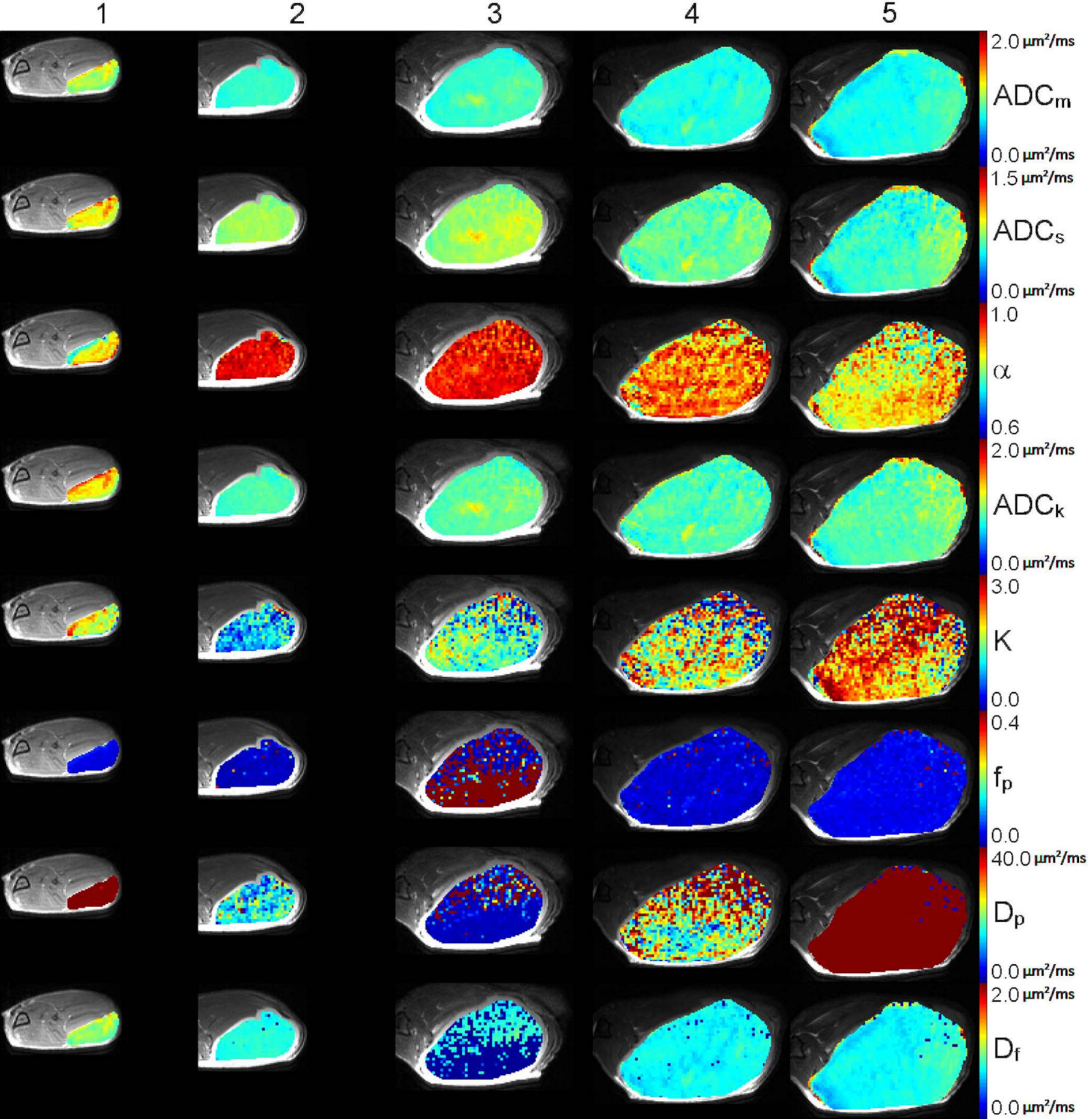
Low b-value DWI data of a representative tumor: T2-weighted image fused with parametric maps for ADC_m,_ ADC_s_, α, ADC_k_, K, f_p_, D_p_, and D_f_ parameters are shown, and represent different degree of tumor homogeneity between week 1 (column 1), week 2 (column 2), week 3 (column 4) and week 4 (column 1). Furthermore, the second repeated imaging on week 4 is shown (column 5). The parametric maps are scaled as follows: ADC_m,_ min−max: 0−2.0 µm^2^/ms; ADC_s,_ min−max: 0−1.5 µm^2^/ms; α, min−max: 0.6−1.0; ADC_k_, min−max: 0−2.0 µm^2^/ms; K, min−max: 0−3.0; f_p_, min−max: 0−0.4; D_p_, min−max: 0−40.0 µm^2^/ms; D_f_, min−max: 0−2.0 µm^2^/ms.
